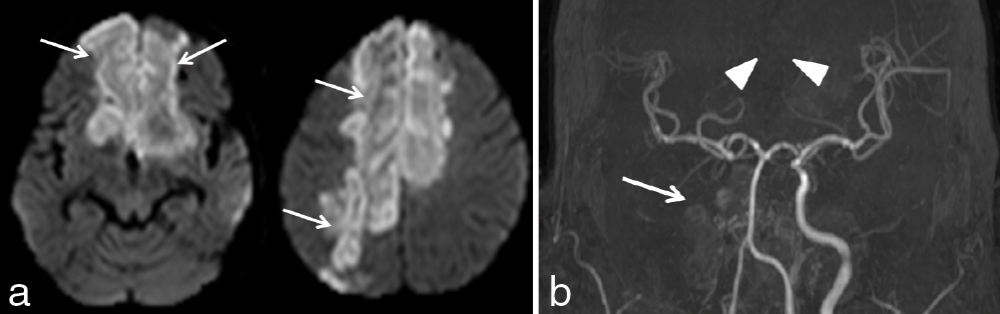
(a, b) MRI repeated after 2 days in view of deterioration despite treatment shows increasing infarcts (arrows in a) and additional thrombosis of bilateral anterior cerebral arteries (arrowheads in b).
